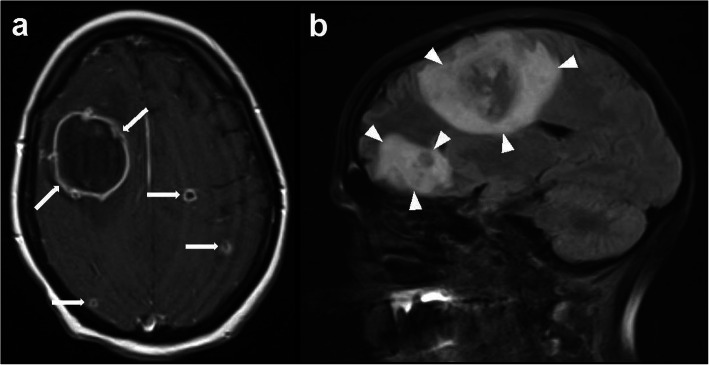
Cerebral MRI reveals multiple mycotic lesions with peripheral contrast media uptake (a: transverse T1 weighted spin echo sequence, *arrows*) and surrounding edema (b: sagittal FLAIR sequence, *arrowheads*) before craniotomy and subsequent abscess removal
